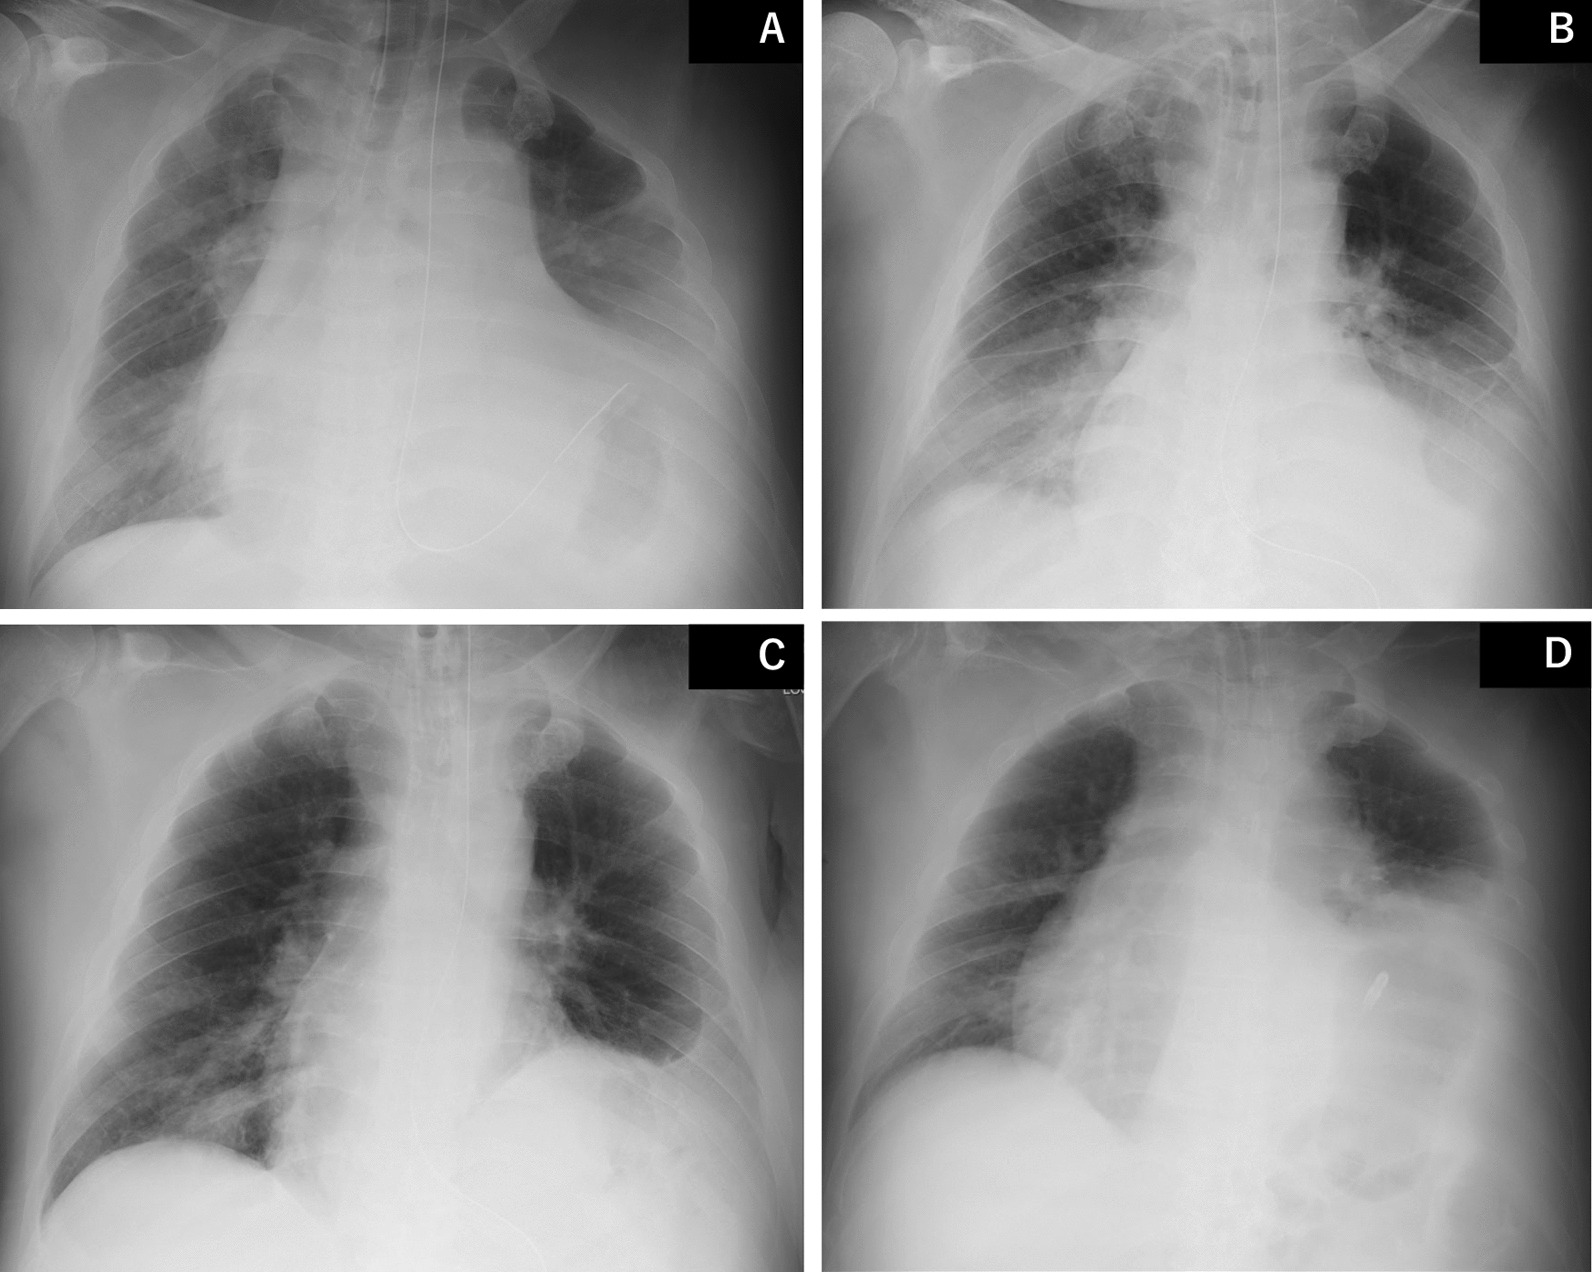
A Chest X-ray on day 2 after bronchoscopy; B Chest X-ray on day 7; C Chest X-ray on day 11; D Chest X-ray on day 29 before ventilator withdrawal
